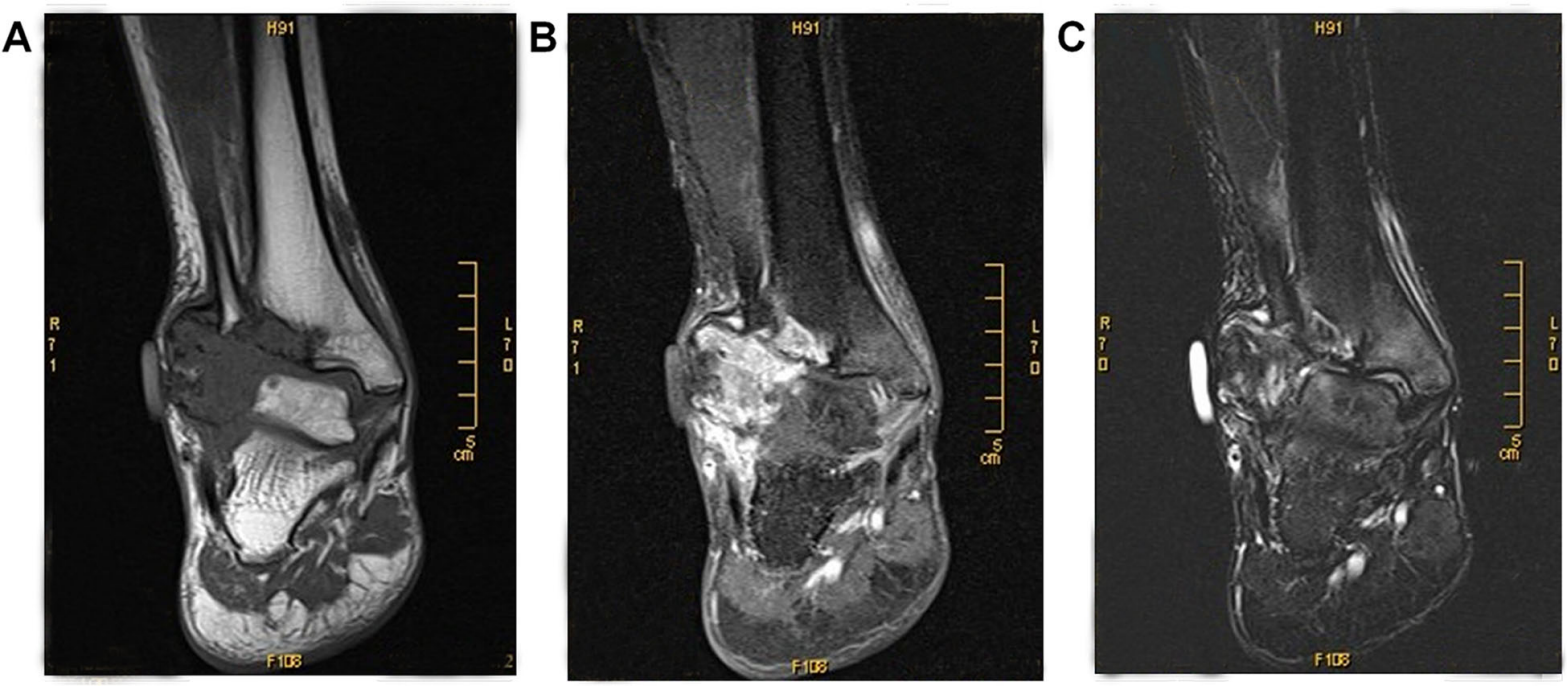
MRI images of massive osteolysis lesion in the right lateral malleolus. a Coronal T1-weighted image shows extensive hypointensity lytic lesions involving the right distal of fibula and partial lateral tibia; b Coronal fat-suppressed T2-weighted images show extensive hyperintensity in lytic lesions; c Coronal T1-weighted images with contrast shows heterogeneous contrast enhancement of the lesion
